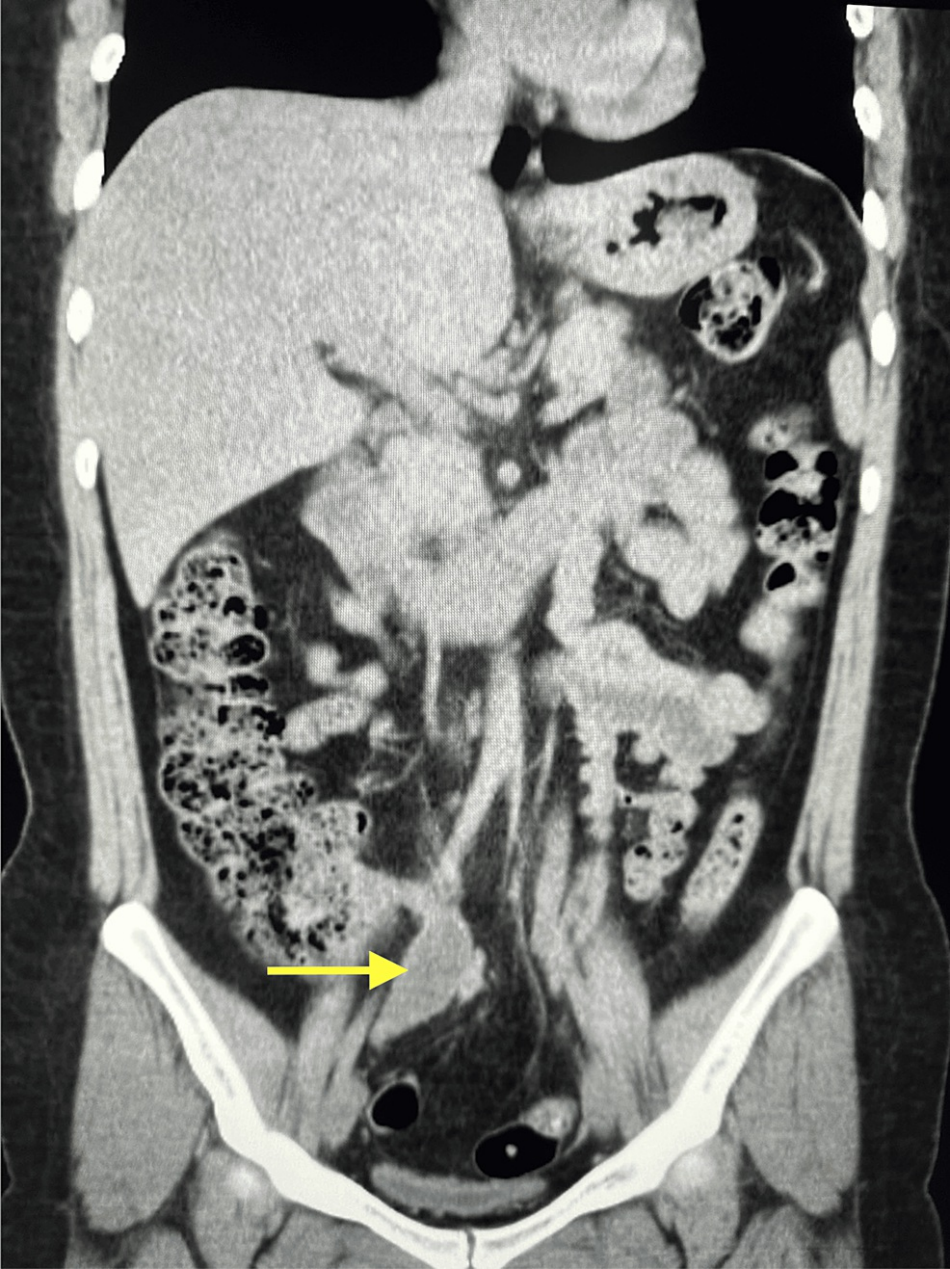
In this coronal CT scan of the abdomen, the yellow arrow points to a 2.3 x 2.4 x 5.2 cm well-circumscribed cystic tubular mass contiguous with the base of the cecum with curvilinear mural calcification suspicious for AMN. CT: computed tomography; AMN: appendiceal mucinous neoplasm
